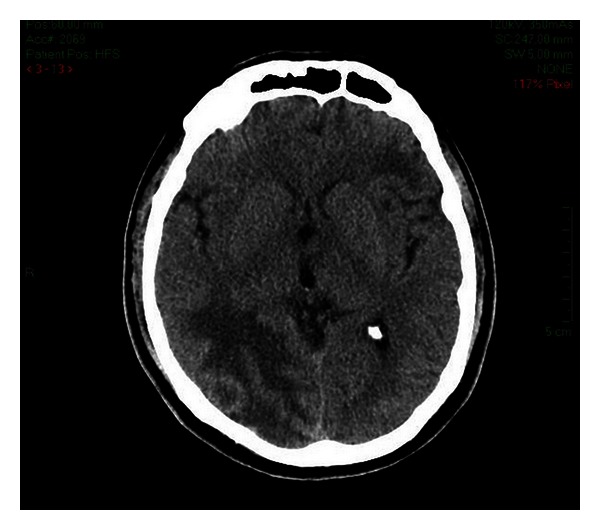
Cystic metastasis. Hypodense, well-demarcated parietal lesion with surrounding digitiform oedema revealed by native CT scan. Department of Neurology, Colentina Clinical Hospital brain imaging archive.
